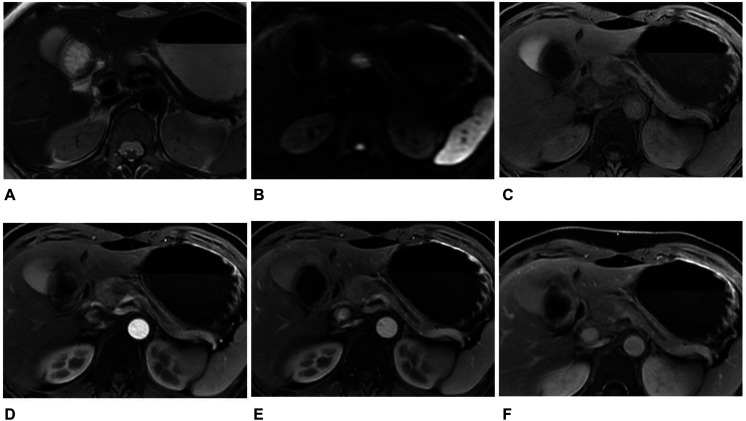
MR images in a 46-year-old woman with an atypical SPT. (A) Axial T2-weighted image showed an ill-defined high signal intensity tumor in the neck of the pancreas, accompanied by distal parenchymal ductal dilatation. (B) DWI with *b* = 1,500 s/mm^2^ showed a hyperintense tumor. (C–F) Axial T1-weighted images obtained during plain (C), arterial (D), portal venous (E), and delayed (F) phases. The tumors showed hypovascular enhancement in the arterial phase, gradual enhancement in the portal venous, and progressive hyperenhancement in delayed phases when compared with the adjacent parenchyma. The diagnosis of pre-operation MRI was PNET. However, the radiomics analysis showed that the tumor was SPT.
